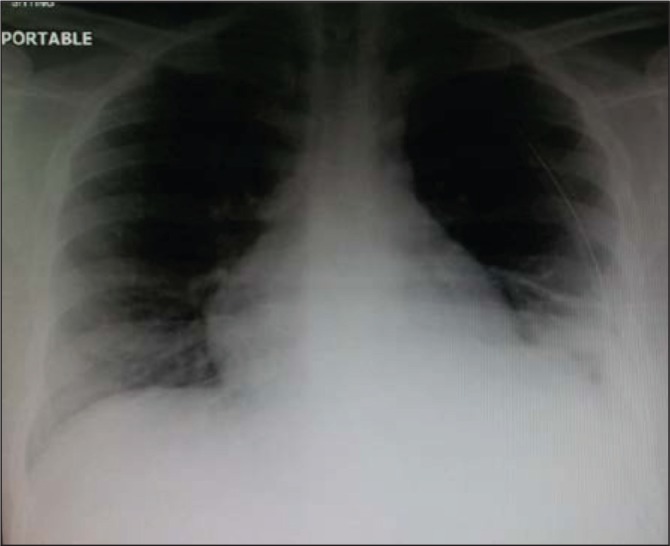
Postoperative chest x-ray showing expansion of the previously collapsed left lung
